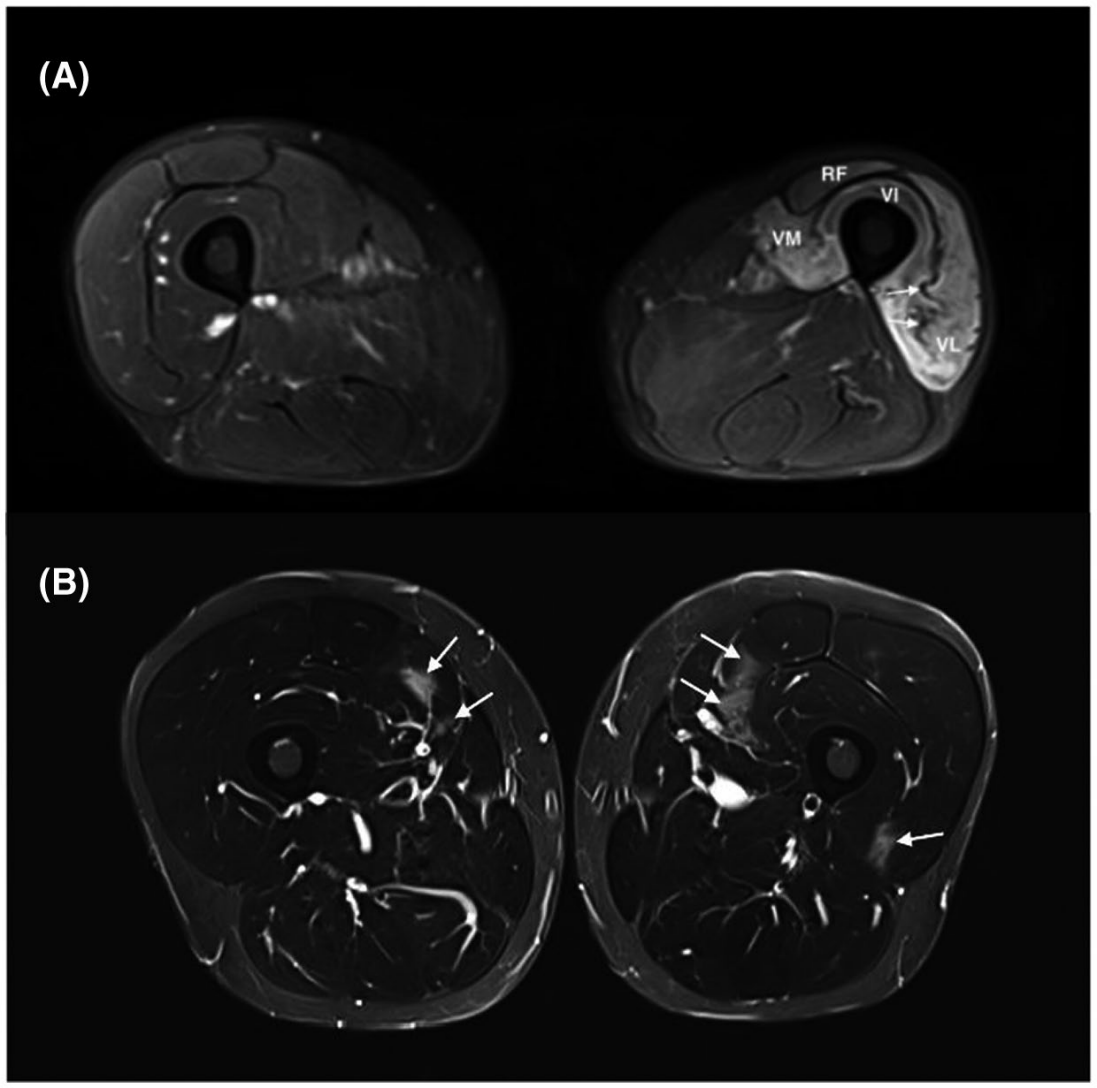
High muscle T2 signals in non‐myositis patients: (A) Neurogenic muscle edema was observed on an MRI performed 4 months after an iatrogenic lesion of the femoral nerve (inguinal hernia surgery). Intense signal and atrophy of the left quadriceps corresponding to the femoral nerve territory are present. Atrophy can be easily identified as compared to the contralateral thigh. Slackness of intramuscular septa (arrow) can also give a clue. (B) MRI 24 h after intense exercise showing patchy areas with increased T2 intensity affecting the quadriceps muscle (arrows), predominantly its medial head, with normal muscle biopsy and spontaneous remission of muscle signs and CK elevation. All pictures show thigh muscles MRI images (axial plane, T2 STIR w. seq). RF, rectus femoris; VI, vastus intermedius; VL, vastus lateralis; VM, vastus medialis
